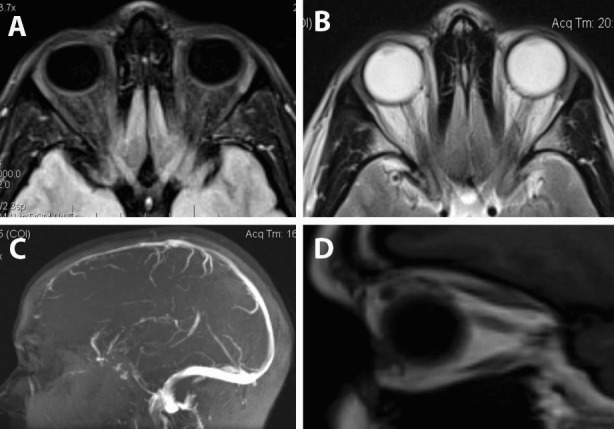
MRI scans of the orbits of the patient. T1-oriented contrast enhanced and fat suppressed scan (A), T-2 oriented scan (B), both at transverse planes. T1-oriented sagittal plane scan (D). MR angiography of the brain (C). All scans are non-contributory concerning orbital or intracranial pathology
